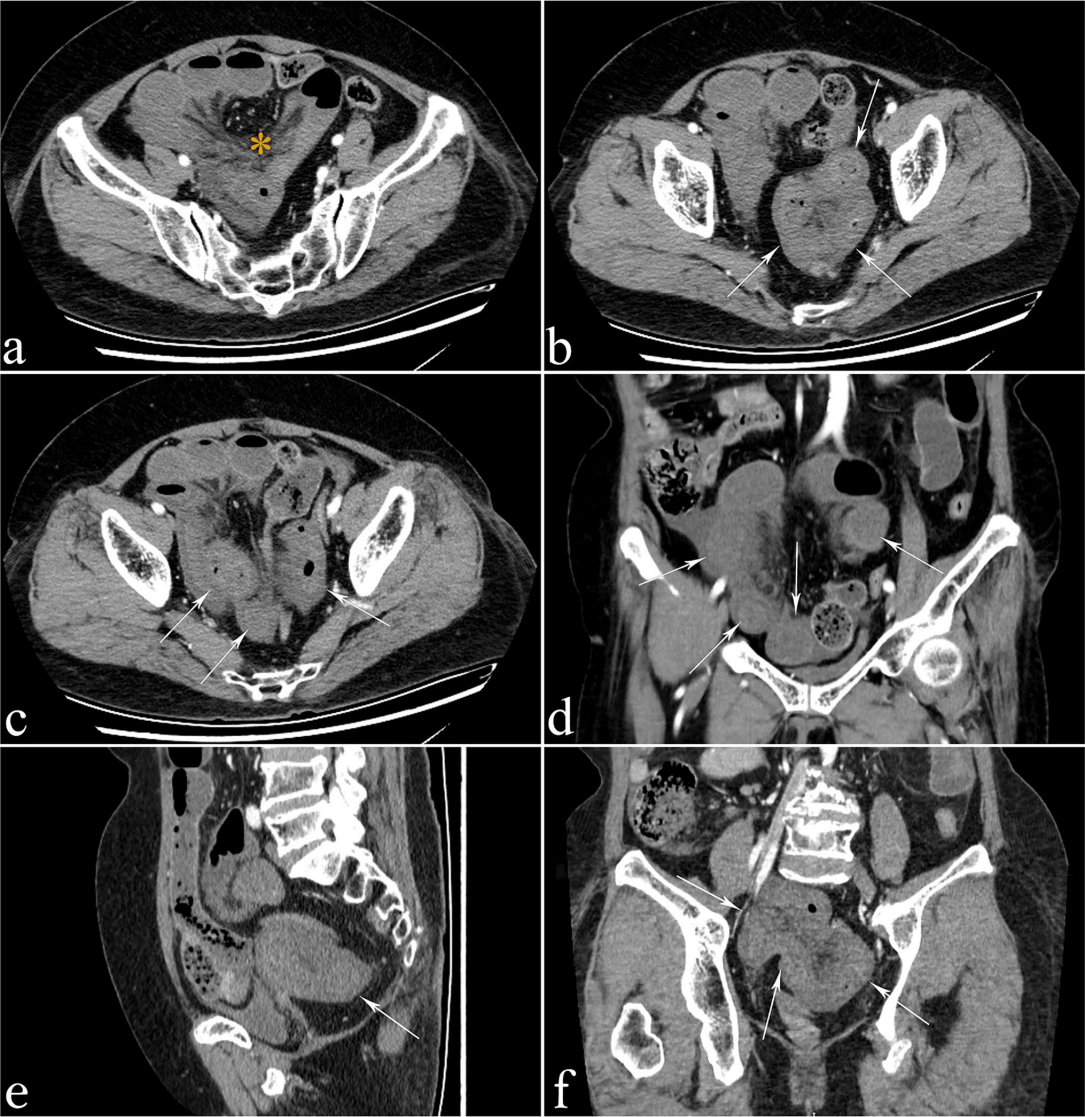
A 59-year-old female patient with adhesive SBO with SBN who underwent appendicitis surgery 2 years ago showed symptoms of abdominal pain and fatigue. a The dilated small-bowel indicates SBO, and mesenteric effusion can be seen (*). b Diffuse thickening and decreased density of the ileal wall with target signs (arrows) are observed, and the CT value of the small-bowel wall is measured to be approximately 15 HU. c After enhanced scanning, the thickening of the ileal wall with the target sign shows a decrease in enhancement (arrow), and the measured CT value of the small-bowel wall is approximately 17 HU, with almost no enhancement. d and e The CT images with coronal and sagittal reconstructions show a thickened ileal wall with the target sign (arrow). f In the image with 3D MPR (Three-dimensional multi-planar reformation), the target sign can be observed (arrow)
